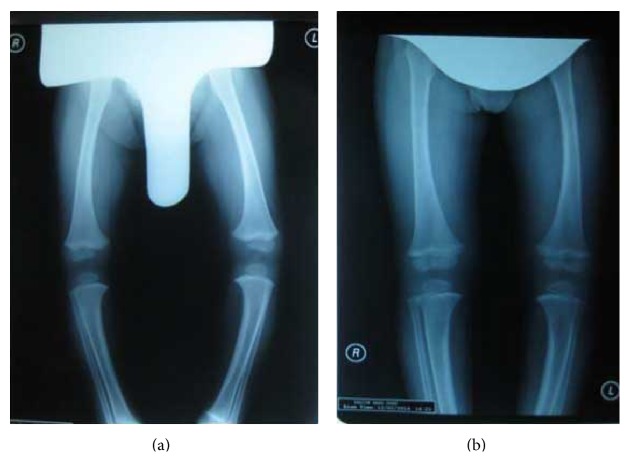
Anteroposterior radiographs of lower extremity: (a) pretreatment, (b) posttreatment.
